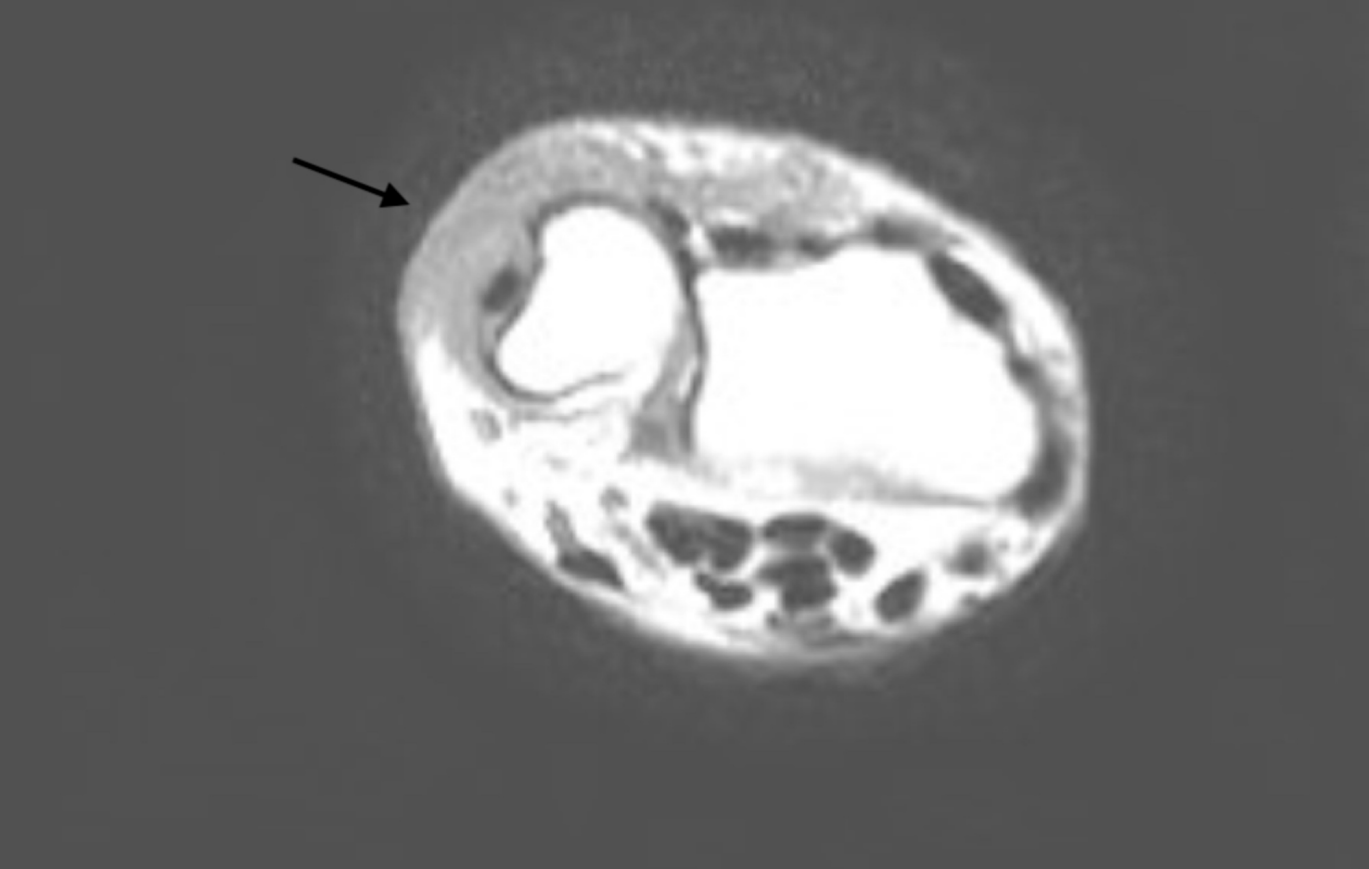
MRI (T1-weighted) of the right arm MRI did not show signs of osteomyelitis, but a signal alteration compatible with corpuscle fluid collection (arrow) could be seen, suggesting a florid activity of infectious inflammatory disease.
